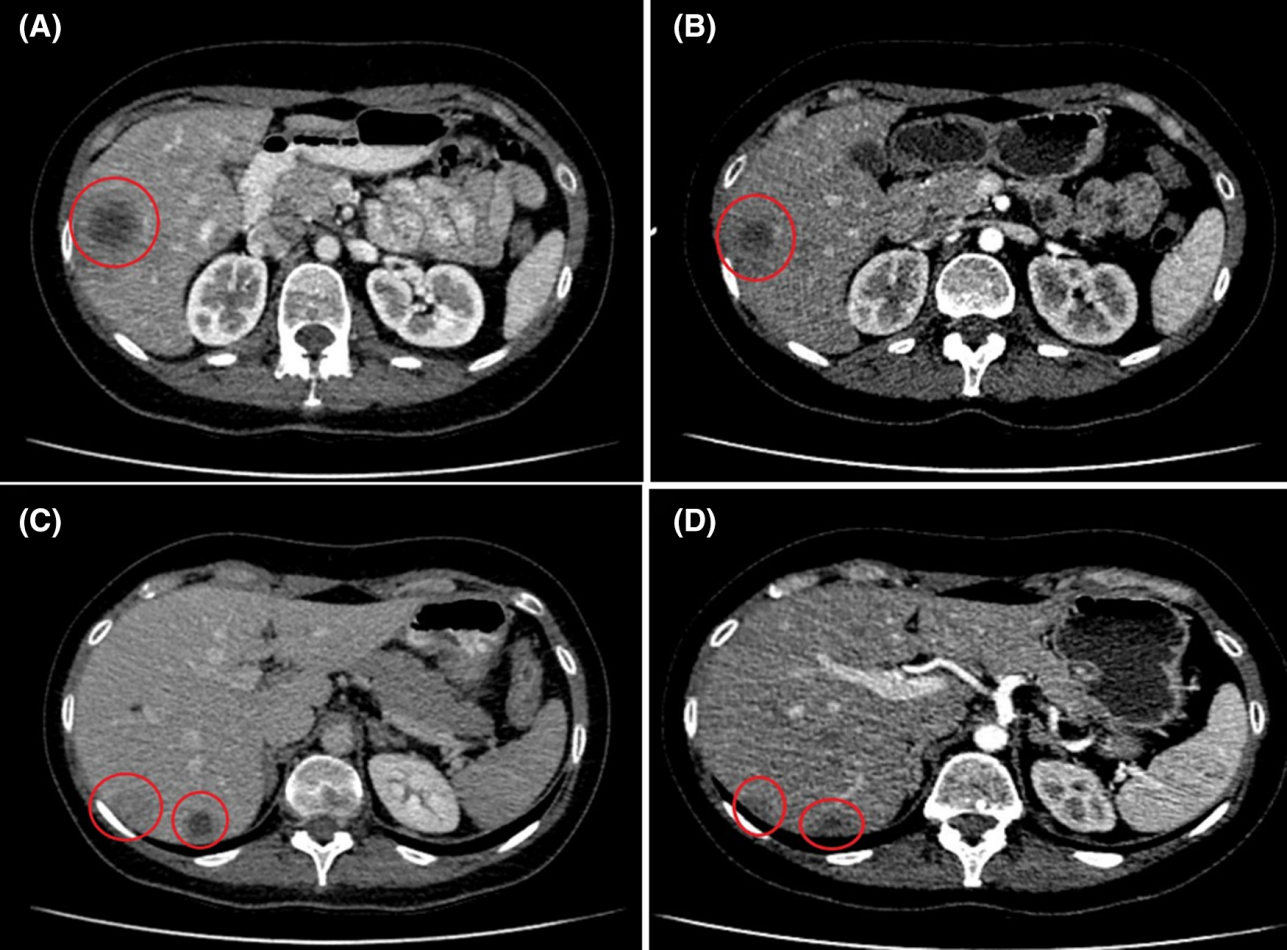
Contrast‐enhanced computed tomography scans of the abdomen showing the metastatic liver lesions before treatment initiation (A, C) and 3 months after (B, D). Red circles highlight the tumor areas.
